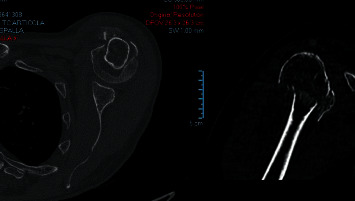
CT scan layer of a PHF in axial and frontal planes.
